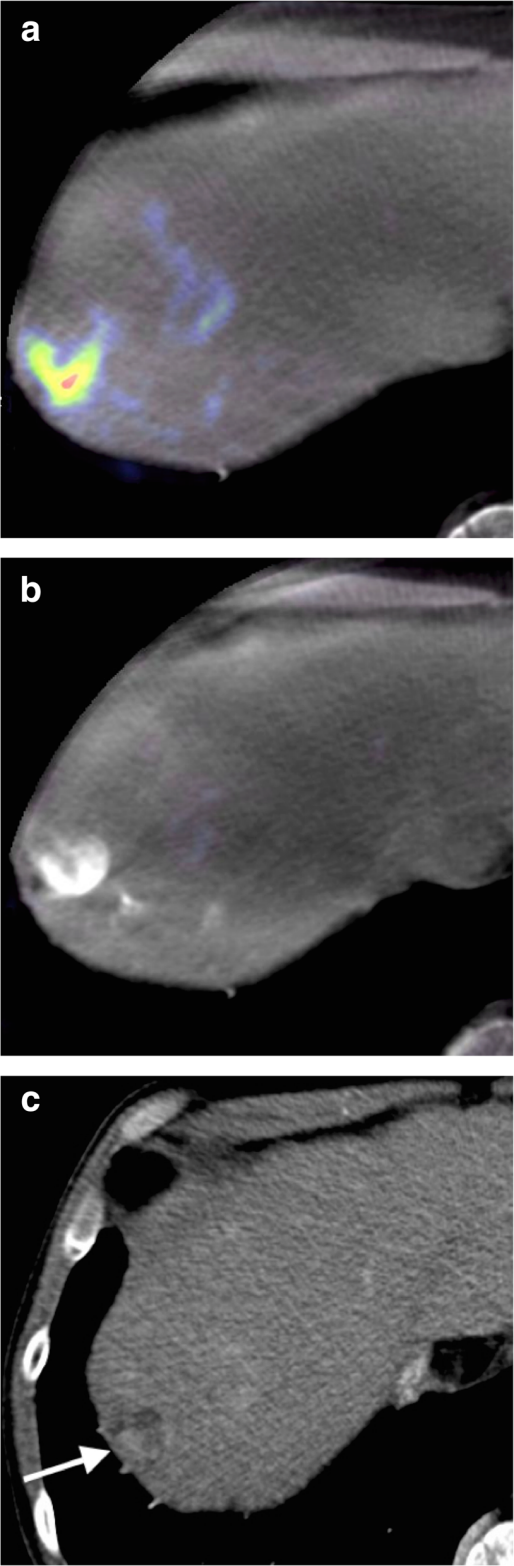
a Pre-TACE C-arm CBCT PBVI via the proper hepatic artery showing viable tumor. TACE has previously been performed. b C-arm CT PBVI via the proper hepatic artery acquired following TACE showing an apparent complete response of the lesion to treatment. There is high density contrast in the lesion but no evidence of residual tumor perfusion. c Follow-up MDCT showing residual enhancing tumor which was subsequently shown to be supplied by the right inferior phrenic artery
